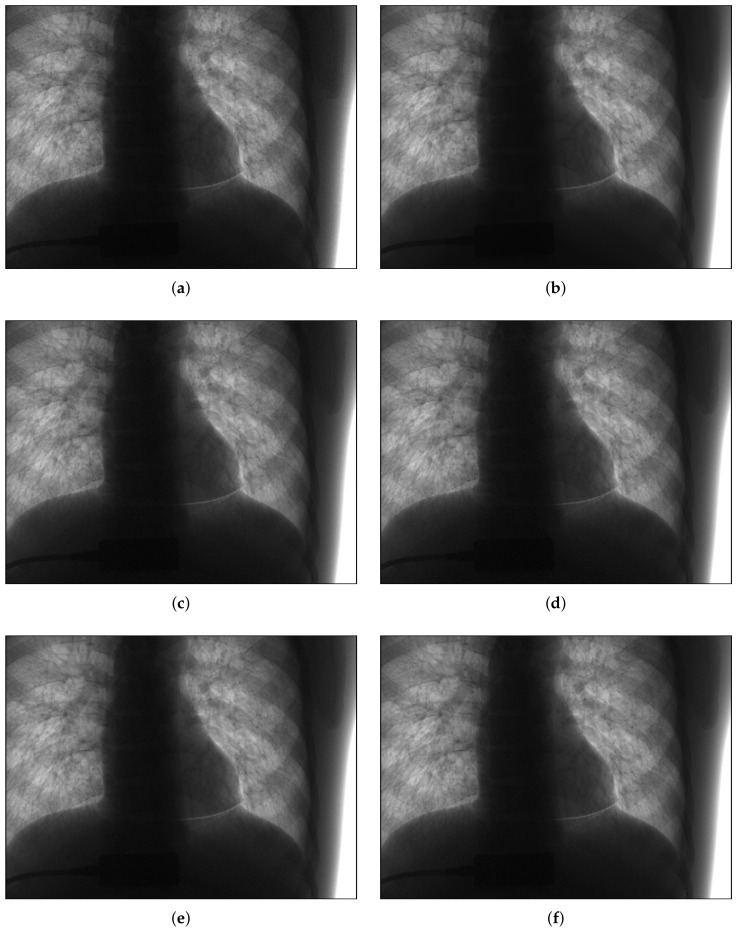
Denoising experiments for real noisy image. (a) low-dose X-ray noisy image; (b) noiseless image; (c–f) denoising results; (c) Du’s method [29] + hard threshold; (d) proposed method + hard threshold; (e) Du’s method [29] + soft threshold; (f) proposed method + soft threshold.
